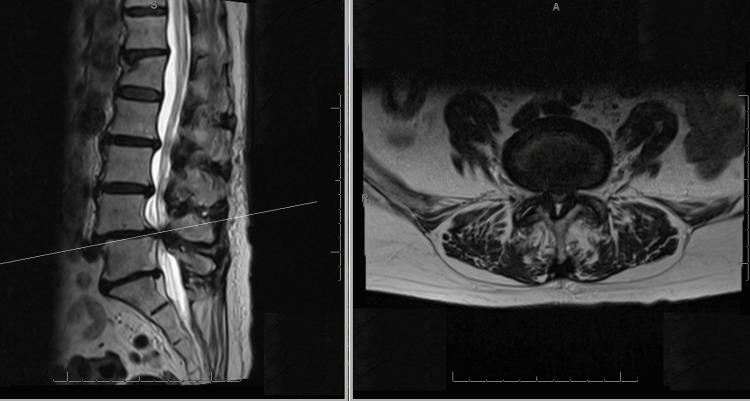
T2-weighted sagittal (left) and axial (right) MRI of the lumbosacral spine showing diffuse degenerative disc disease. There is marked central canal stenosis at the L4-5 level due to ligamentum flavum hypertrophy, with ligamentum flavum measuring 3.9 mm.
